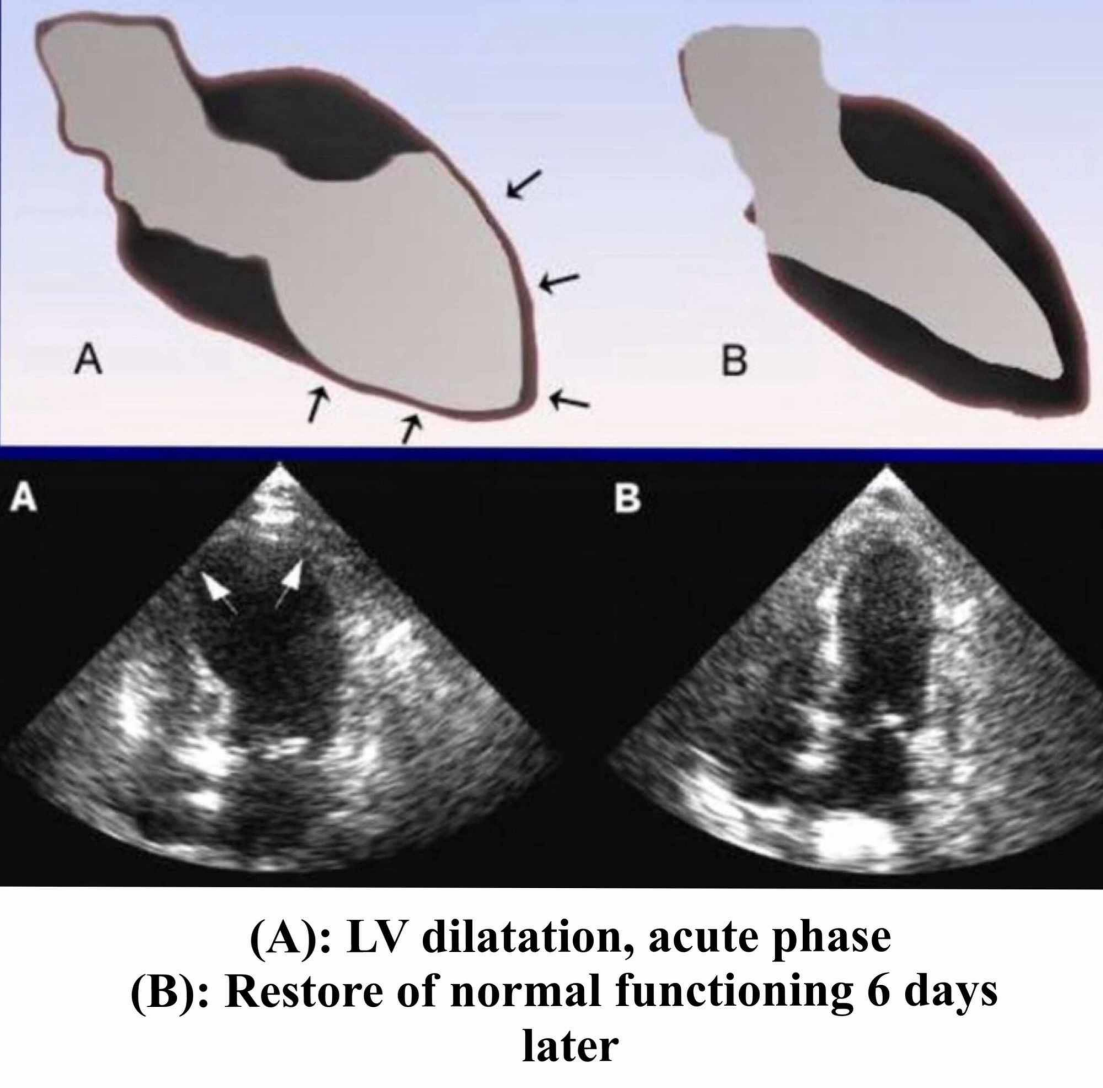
Echocardiographic changes in TTC TTC: Takotsubo cardiomyopathy
